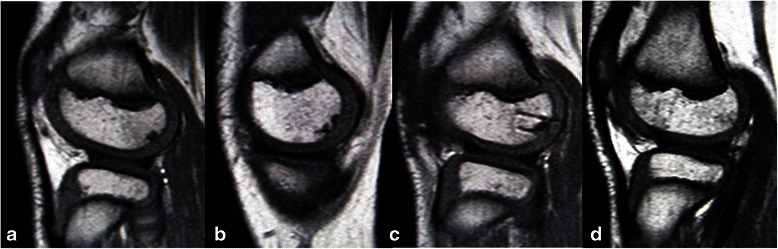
T1-weighted sagittal magnetic resonance imaging images at 1 year and 3 months after the second surgery. a Lateral femoral condyle of the patient’s right knee following transchondral drilling. b Medial femoral condyle of his right knee following transchondral drilling. c Lateral femoral condyle of his left knee following cylindrical osteochondral grafting. d Graft donor site in the lateral femoral condyle
